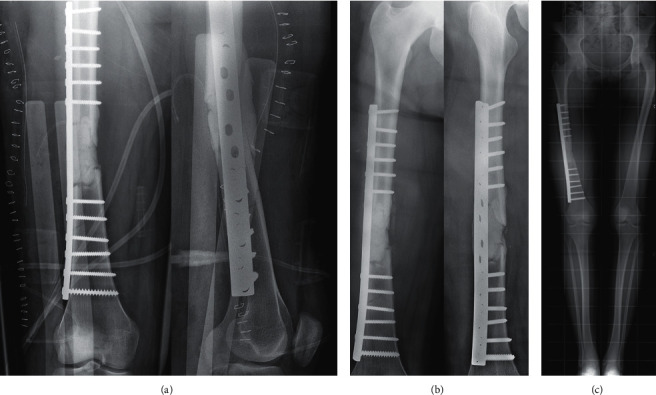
After a surgical debridement comes the first stage of the modified Masquelet technique. (a) The large defect was filled with cement, and the fracture was stabilized with a 4,5 mm Dynamic Compression Plate. (b) Follow-up views at 4 weeks post first stage. (c) At the full-length weight-bearing (FLWB) X-ray, there was no leg length discrepancy.
